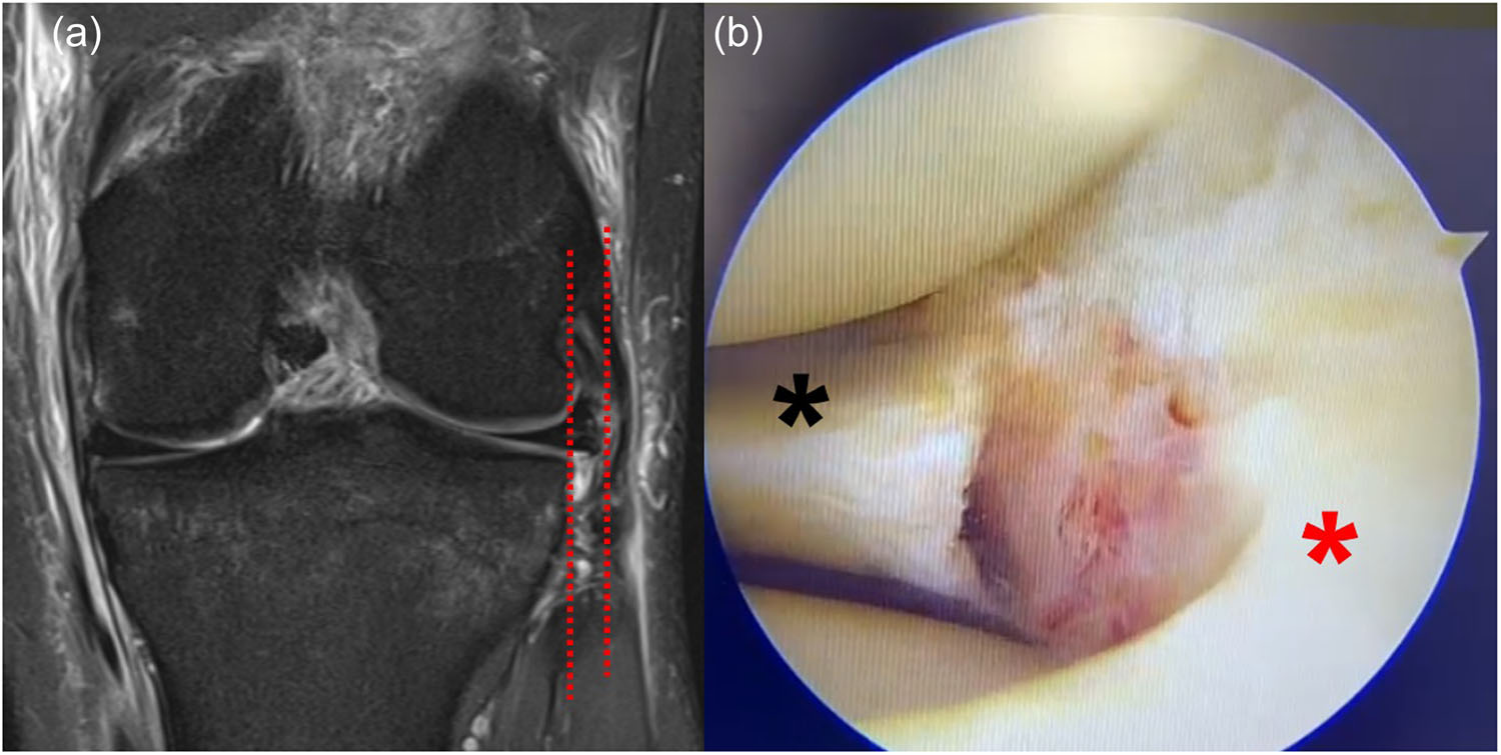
(a) MRI coronal view of a left knee. The red‐dotted lines represent the amount of meniscal extrusion. (b) intraoperative arthroscopic view of the radial lateral meniscal lesion, the red and black asterisk pointed out the two meniscal segments. MRI, magnetic resonance imaging.
